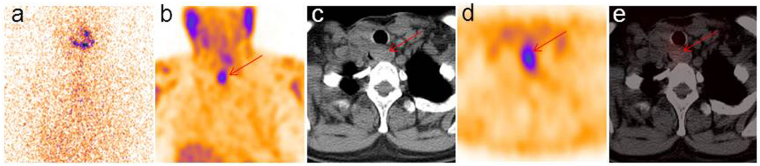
Typical SPECT/CT images of focal uptake patterns. ^99m^Tc-3PRGD2 imaging was performed 1 week after the negative diagnostic dose ^131^I WBS (a), to establish the presence of recurrence and/or metastatic disease in a TENIS syndrome patient. ^99m^Tc-3PRGD2 MIP SPECT image showed focal uptake in thoracic region (b), following SPECT/CT revealed increased radiopharmaceutical uptake corresponding to the enlarged lymph nodes (c–e, arrows).
